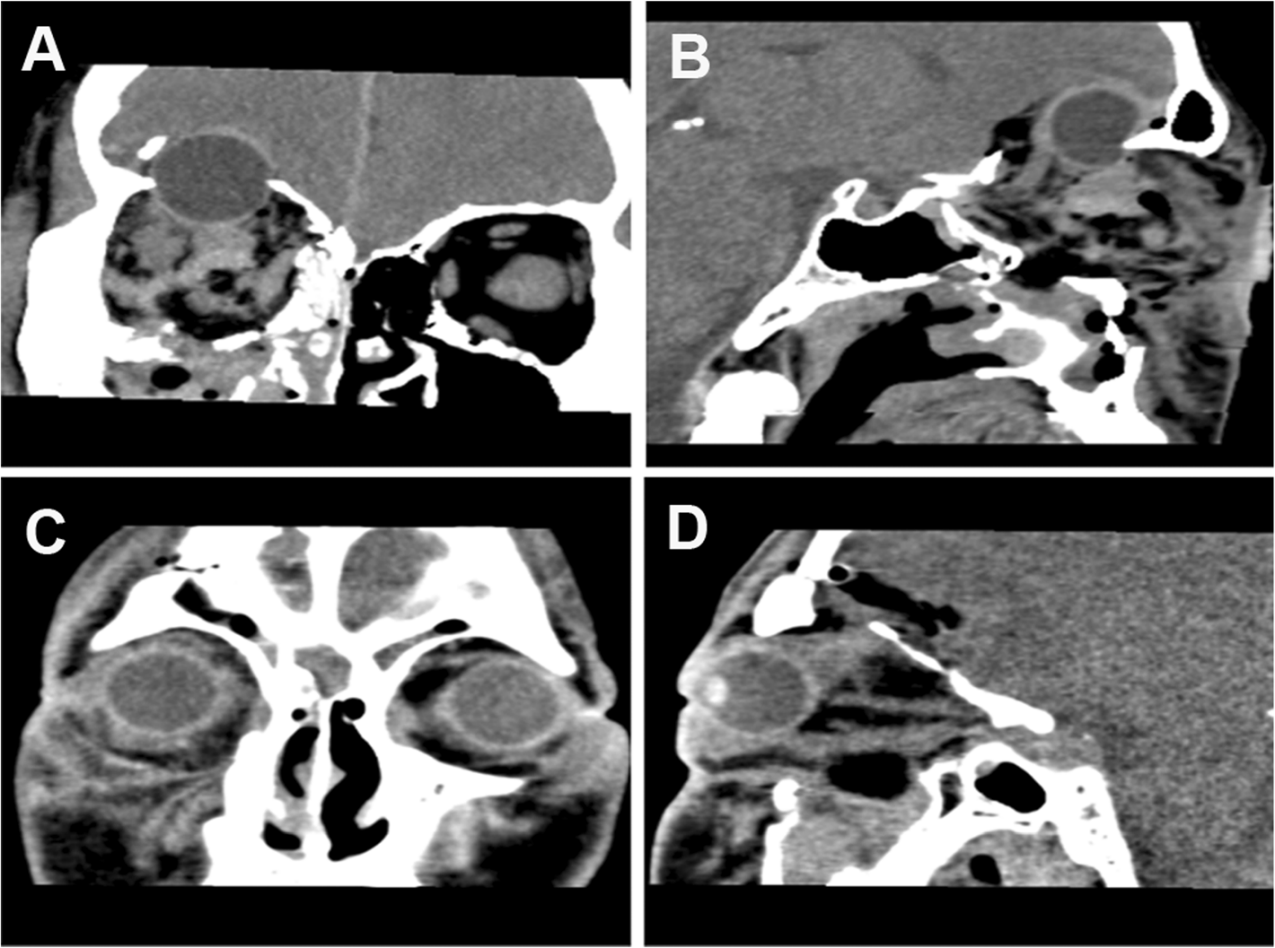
a Coronal CT scan showing large fractures of the right orbital roof and incomplete dislocation of the right globe into the anterior cranial fossa. b Sagittal CT scan showing that no fractures were noted in the optic canal, but that the optic nerve appeared kinked. c Coronal CT scan showing that the dislocated globe was repositioned to its correct anatomical position on postoperative day 1. d Sagittal CT scan showing the continuity of the optic nerve on postoperative day 1
